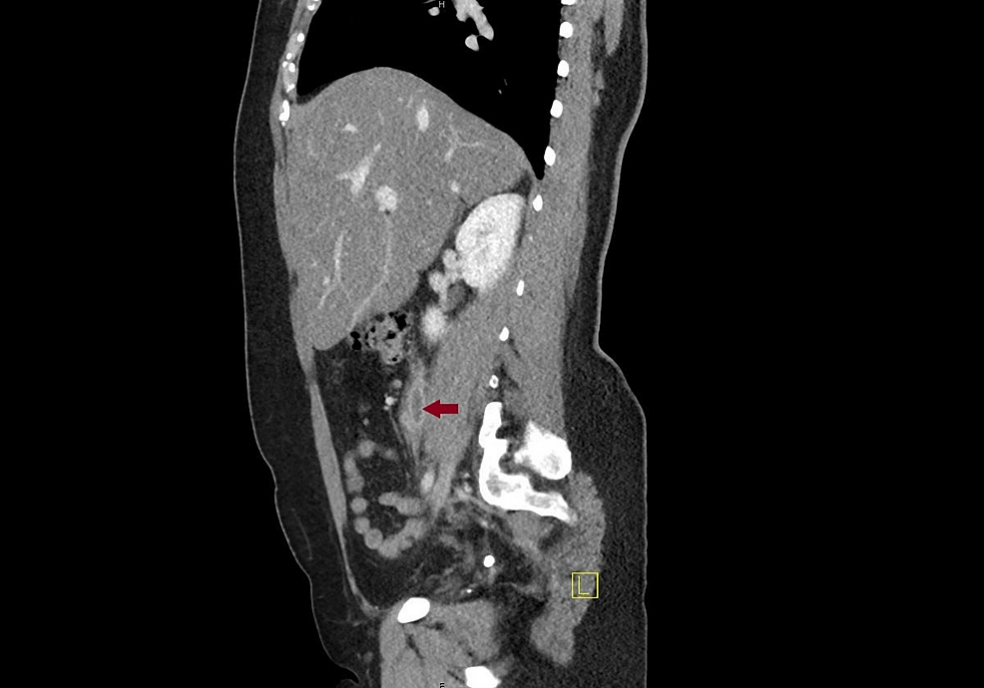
Sagittal view of computed tomography scan with contrast of abdomen and pelvis showing right ovarian vein thrombosis (Arrow)
